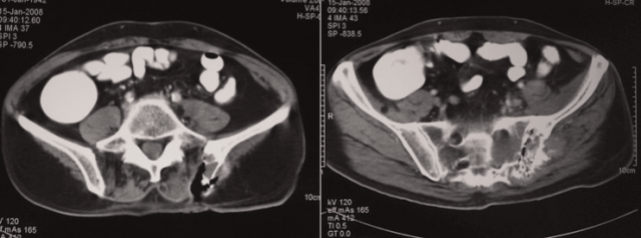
Follow-up CT scan images 3 months post procedure show a large tissue deficit corresponded to ablated tissue. Note the air within cavitary lesion.
